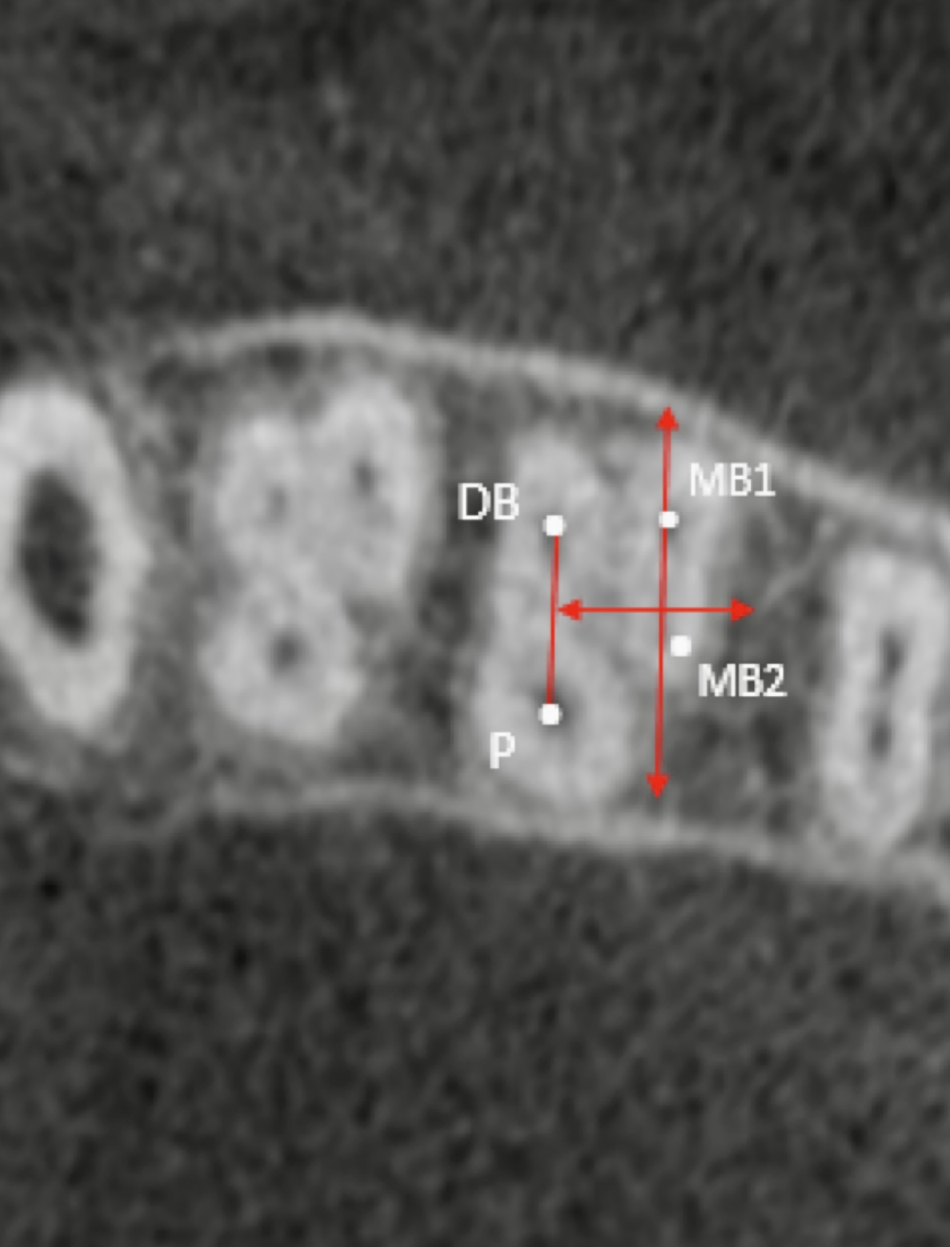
Representative CBCT scan of the evaluated mean distance between the main mesiobuccal and second mesiobuccal canal orifices.
